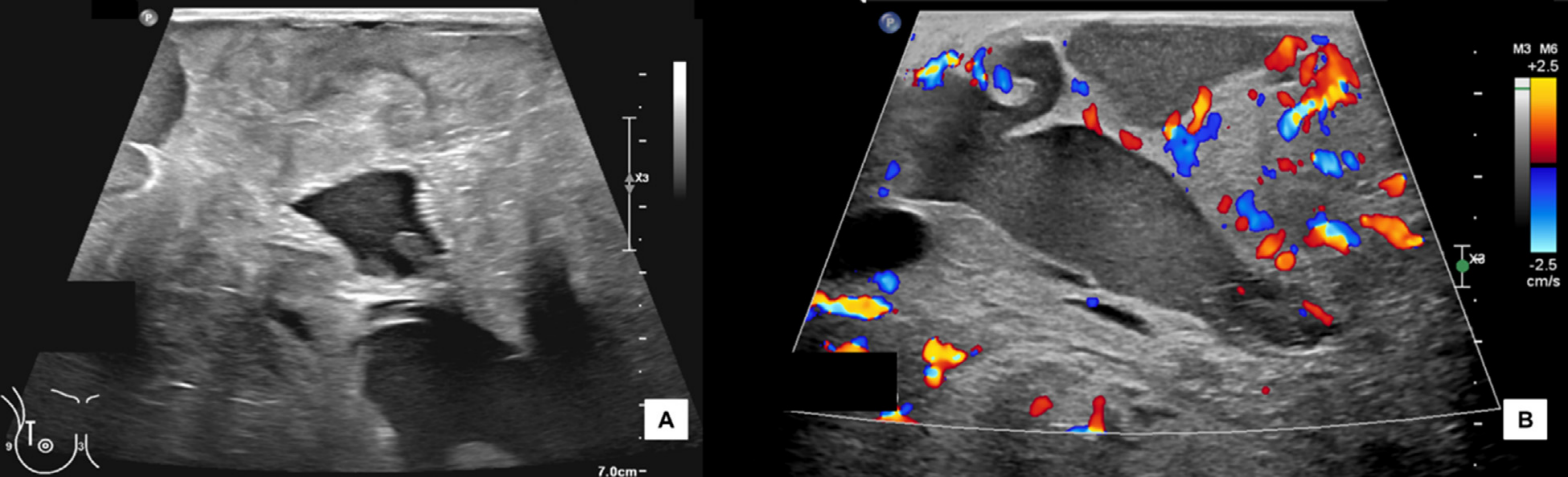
(A) Ultrasound of the right breast demonstrating the large heterogenous mass with cystic spaces in the upper outer quadrant and (B) color Doppler ultrasound showing internal vascularity in the upper inner quadrant of the mass.
